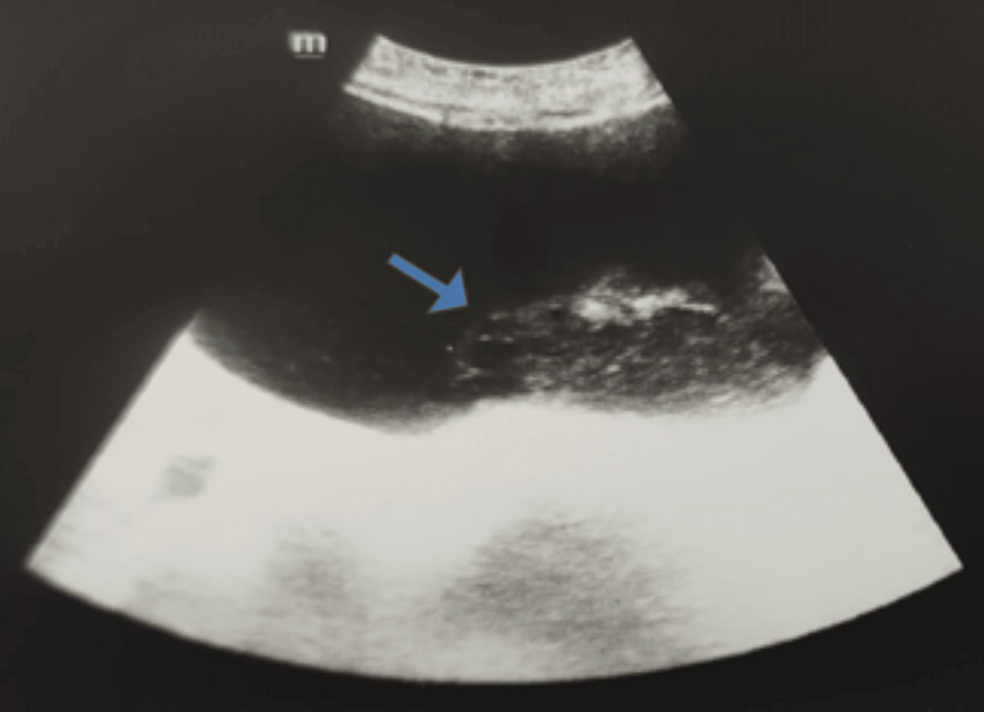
A fluctuating lesion with heterogenous echo 4 x 2 cm in size was detected on ultrasound
